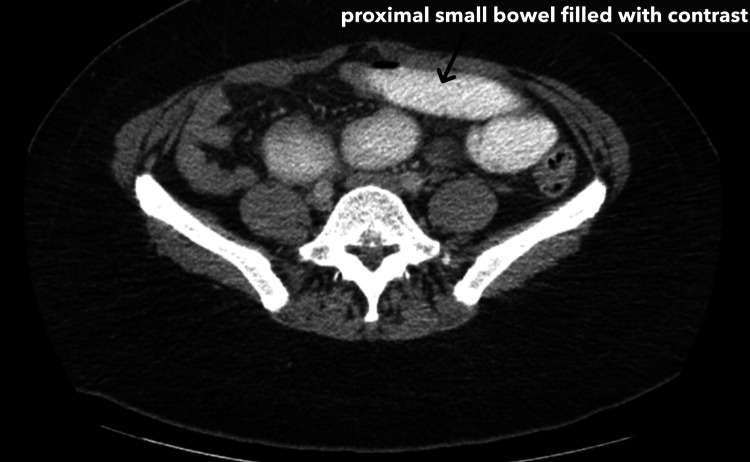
CT scan findings of the dilated, contrast-filled small bowel proximal to the hernia site The axial slide of the CT with oral contrast shows a dilated, contrast-filled portion of the small bowel, which is proximal to the site of the hernia. The proximal dilation indicates obstruction of the small bowel at the site of the hernia.
